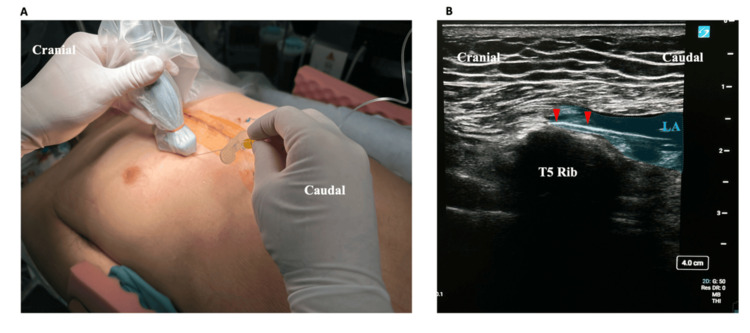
Superficial parasternal intercostal plane block (SPIPB) procedure. (A) Probe placement in the sagittal orientation. (B) Ultrasound image showing local anesthetic (LA) spread beneath the pectoralis major muscle (PMm). The block needle was advanced from the caudal to the cranial direction using the hydrodissection technique. The red arrowheads indicate the needle trajectory along the T5 Rib.
